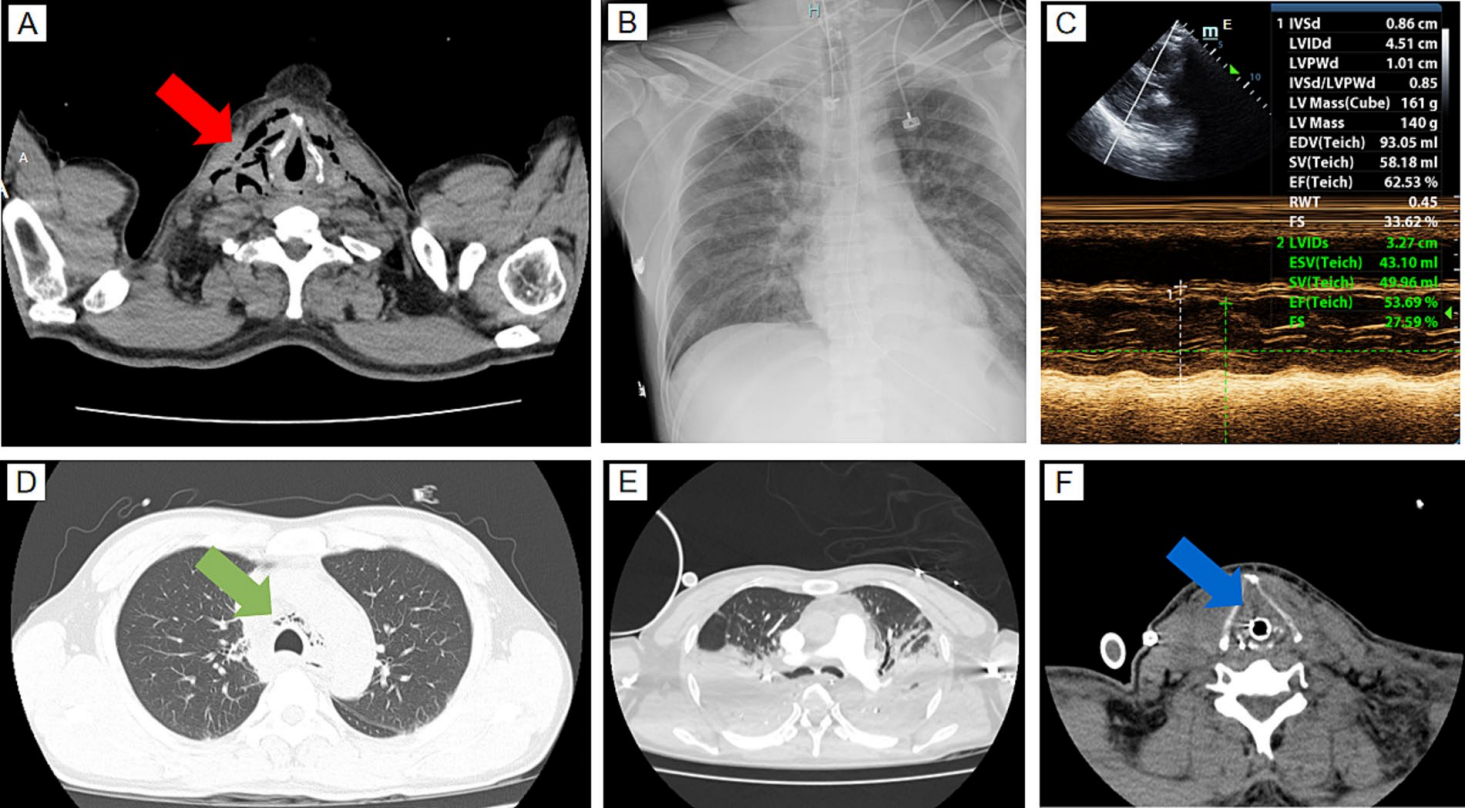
Imaging studies at admission and prior to ECMO initiation. (A) Chest CT scan at admission showing subcutaneous emphysema in the neck region (red arrow). (B) Chest X-ray before ECMO initiation showing diffuse bilateral lung shadows, indicating severe lung involvement. (C) Echocardiographic assessment before ECMO initiation showing an ejection fraction (EF) of 62.53%. (D) Chest CT scan at admission demonstrating mediastinal emphysema (green arrow). (E) Contrast-enhanced chest CT after ECMO initiation revealing worsening lung infection with consolidation. (F) Oropharyngeal CT scan after ECMO initiation showing neck soft tissue swelling and compression of the airway surrounding tissues (blue arrow).
